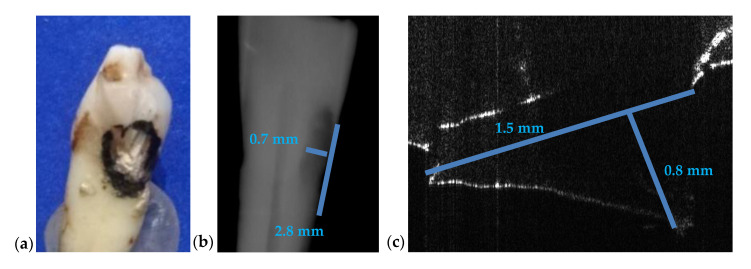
(a) Incisive tooth with an area marked for OCT investigations; (b) a section cropped from the intraoral radiography with measurements of the cavity; (c) OCT B-scan where the depth and the length measurements of the cavity have been performed.
